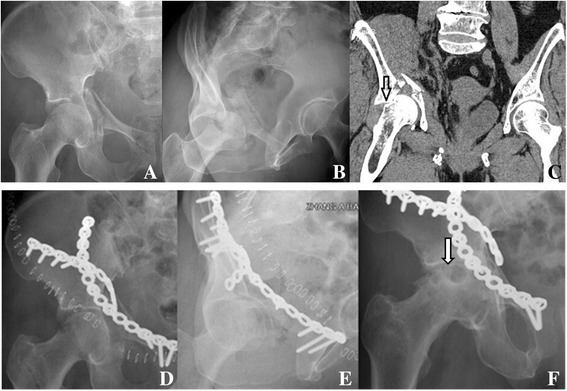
Radiographs of a 66-year-old female patient with an anterior wall acetabular fracture. a Anteroposterior radiograph; b Obturator oblique radiograph; c CT scan, showing femoral head injury (arrow). d, e Immediately after surgery, showing a satisfactory reduction of fracture; f The radiograph taken at 30 months postoperatively, showing an osteonecrosis of the femoral head (arrow)
